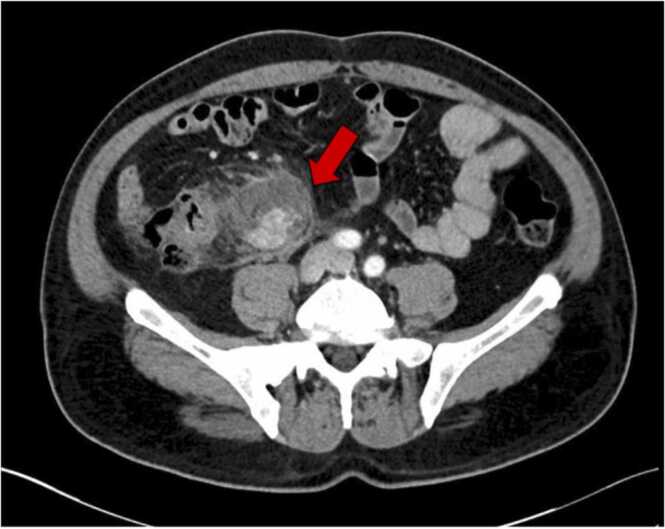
Initial CT abdomen/pelvis. Red arrow indicates an ill-defined 5–6 cm focal fluid collection with surrounding inflammation in the right mid abdomen center around what appears to be a ruptured aneurysm or pseudoaneurysm.
